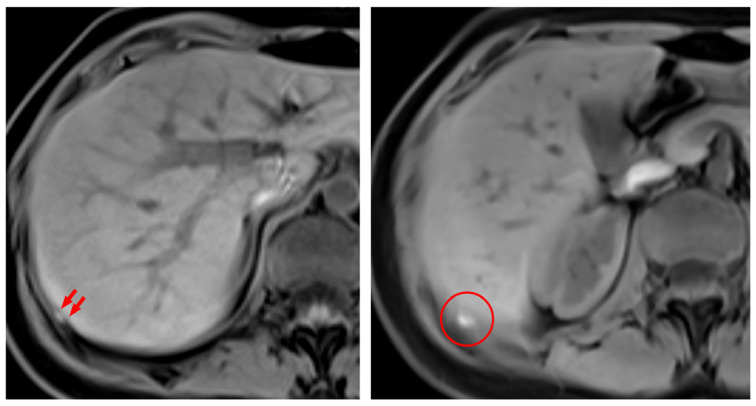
Diaphragmatic endometriosis in a 28-year-old woman. Axial fat-saturated three-dimensional T1-weighted MRI shows high signal intensity nodules attached to the posterior part of the right hemidiaphragm. The red arrows (on the left) point to the endometriotic nodule and the red circle (on the right) demonstrates the location of the endometriotic nodule with the lesion being in the middle.
